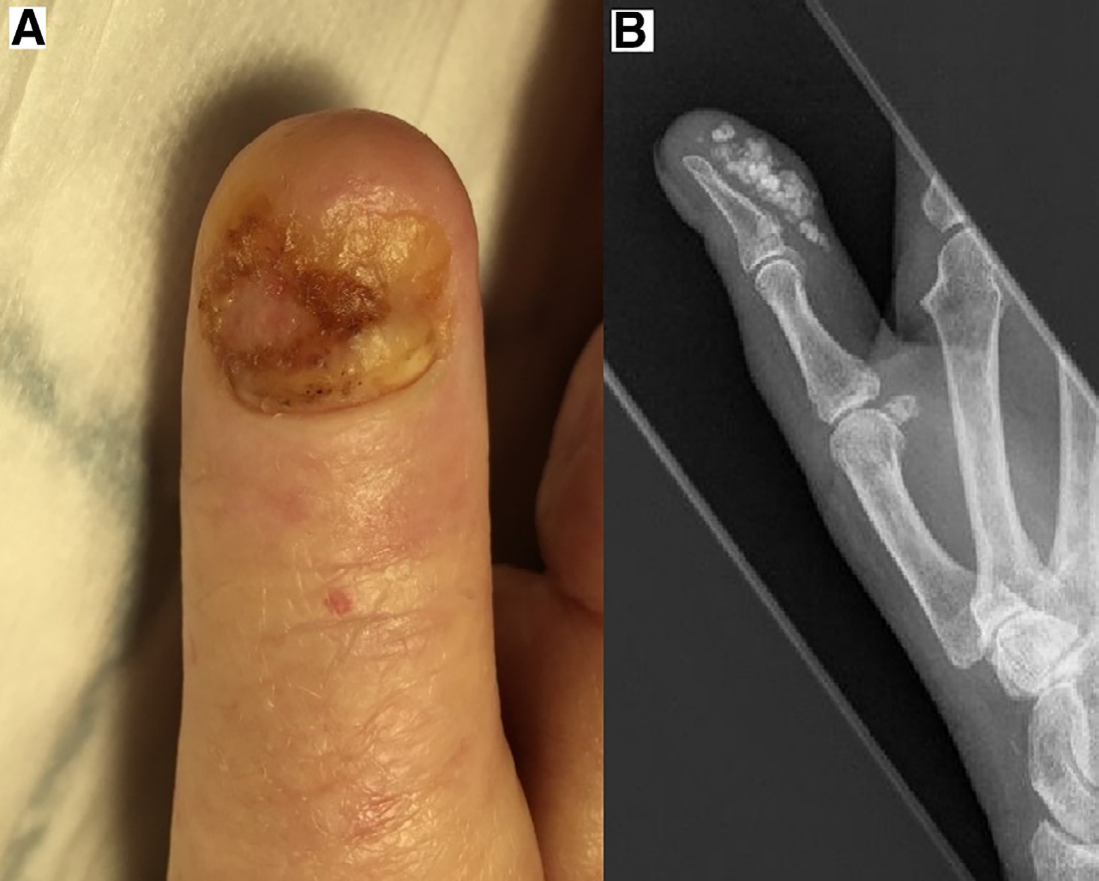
(A) Showing right thumb nail bed SCC. (B) Radiograph of right thumb showing extensive subcutaneous calcinosis.
